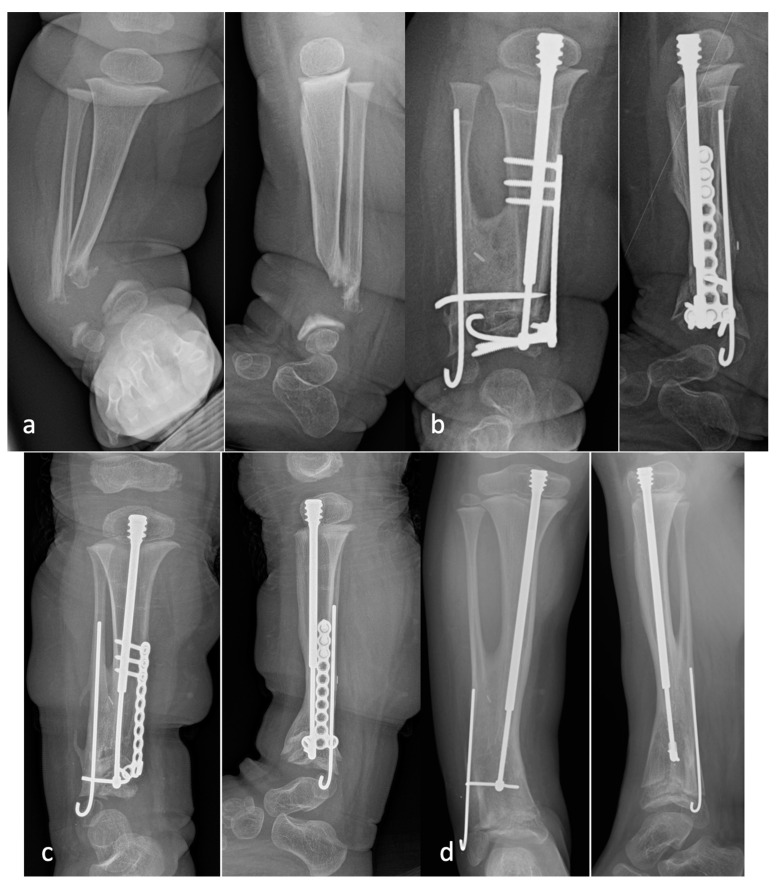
Radiographs of a patient who underwent a cross-union with internal fixation: (a) preoperative AP and lateral radiographs demonstrating a large bone defect and small distal metaphyseal segments of the tibia and fibula (Type 4C); (b) AP and lateral radiographs taken 12 weeks after surgery show a well-healed cross-union with bridging between the tibia and fibula, as well as filling of the bony defects; (c) AP and lateral radiographs taken 1 year after surgery with growth of the bone at both ends and telescoping of the FD rod to the level of the cross-union; (d) AP and lateral radiographs taken at 2.5 years after surgery, after a one-rod exchange, with a solid cross-union and no refracture.
